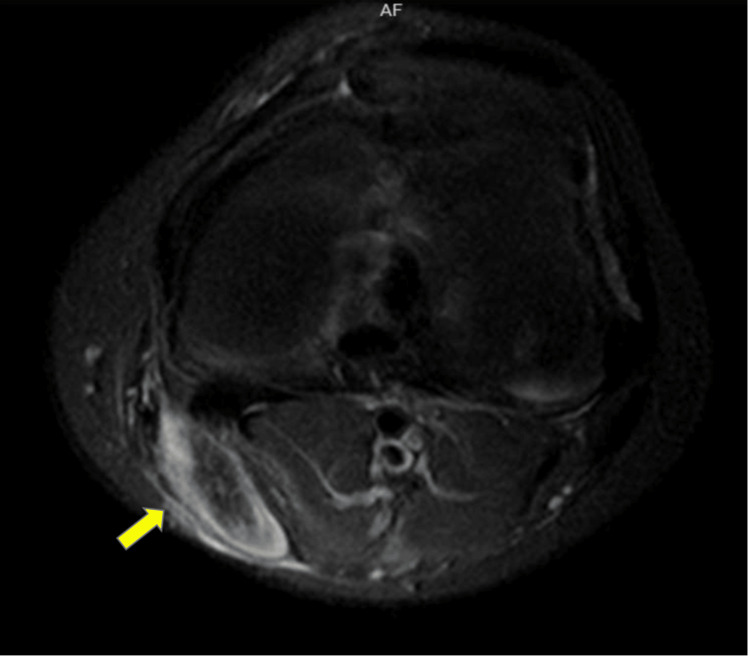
Axial short-T1 inversion recovery magnetic resonance imaging: Left knee level. High signal fluid collection (yellow arrow) surrounding the thickened ruptured semitendinosus tendon, extending into the medial aspect of the upper calf muscle region.
